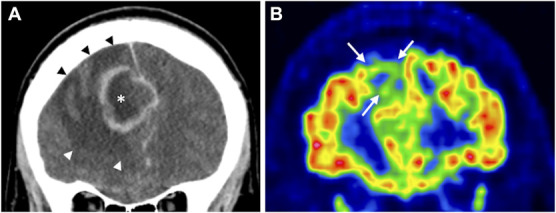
Neocortical hypometabolism 9 years after brain abscess. A, Coronal CT scan showing an abscess (*) in the right frontal lobe causing midline shift before pus removal. Note peri-abscess vasogenic edema (black and white arrowheads). B, [^18^F]-fluoro-2-deoxy-D-glucose positron emission tomography/CT (coronal section) of the same patient 9 years after pus removal, showing widespread neocortical hypometabolism in the area of the previous abscess (arrows). CT, computed tomography.
